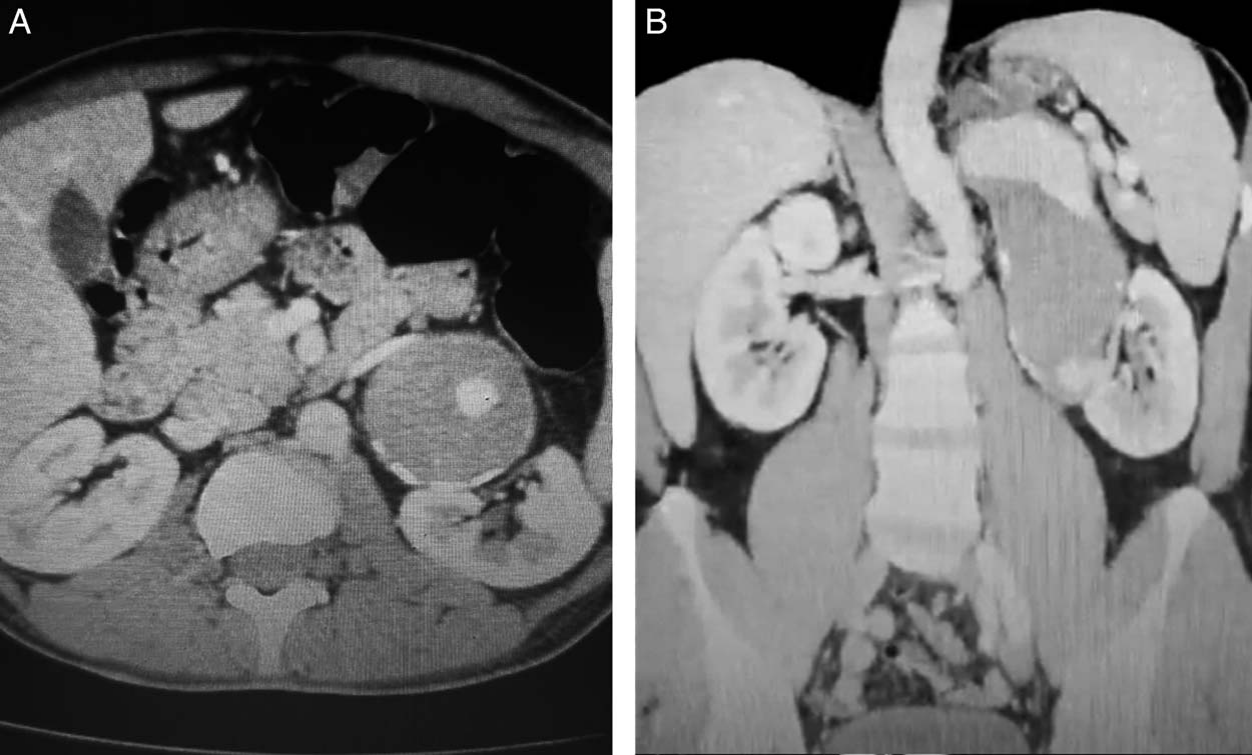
(A) MSCT with contrast (transverse view): a giant left renal aneurysm compared to the aorta, compressing the left renal vein and dislocating the left kidney downward. (B) Multi-slice computed tomography (MSCT) with contrast (coronal view): true left renal artery aneurysm (12.5 ×9 cm), true lumen (4.5 ×3 cm).
